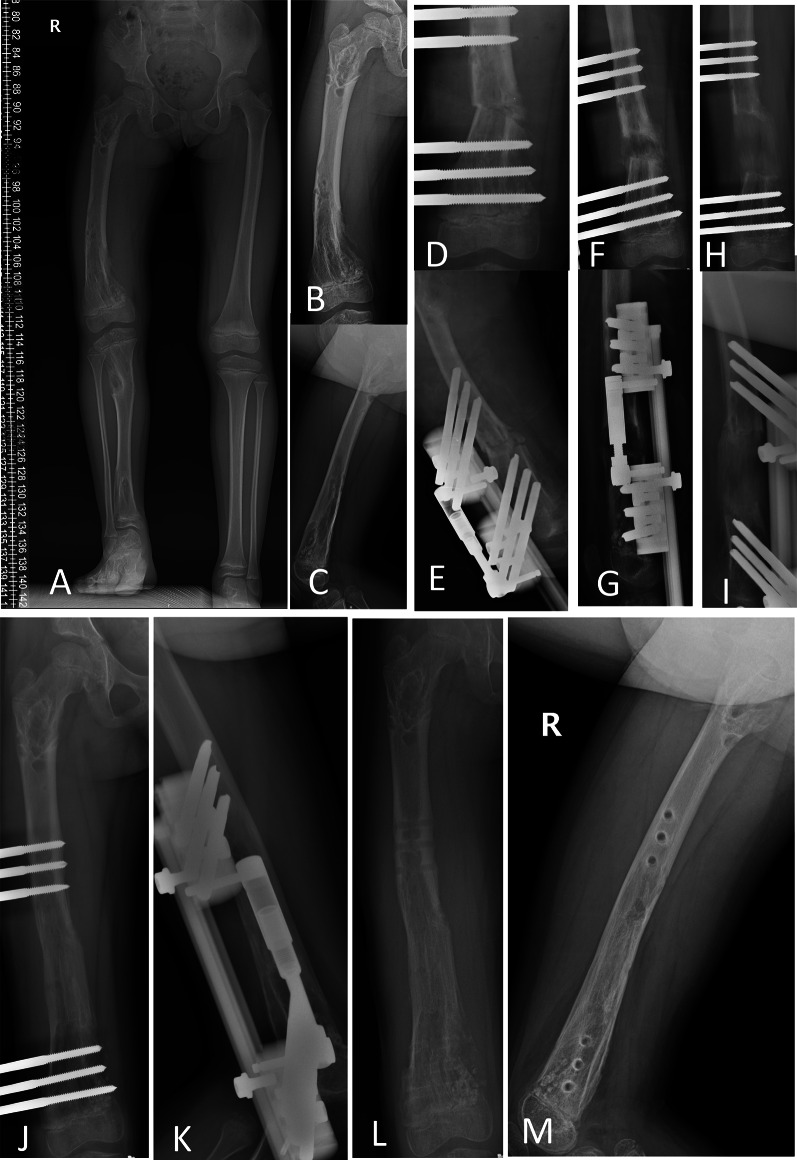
A 4Y10M girl (patient 2) with Ollier’s disease, right femur lengthened by 5.90 cm, 16.4°AC, with OrthoFix fixator. A Preoperative full-length AP. B, C AP and lateral radiographs before operation. D, E AP and lateral radiographs during operation. F, G AP and lateral radiographs after 30 days of lengthening. H, I AP and lateral radiographs at the end of lengthening after 61 days. J, K AP and lateral radiograph before external fixation removal, 6 months after operation. L, M AP and lateral radiographs 1 month after removal of the external fixator
